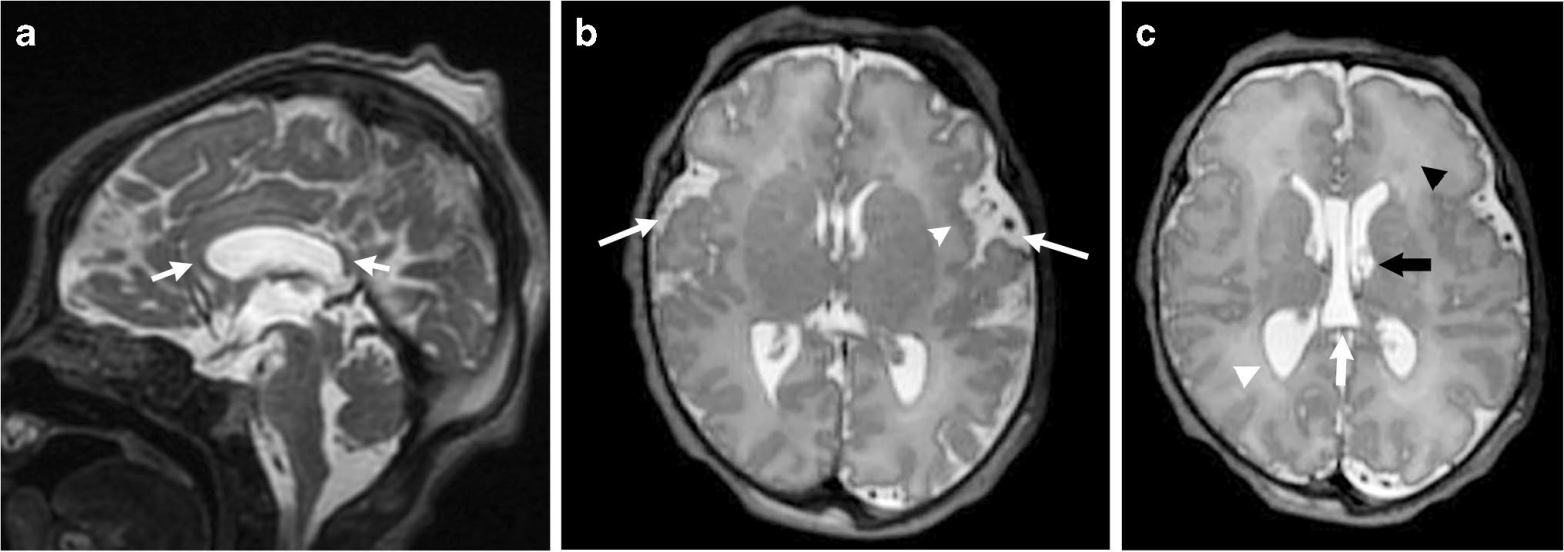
Sotos syndrome in a 4-day-old boy with genetically confirmed *NSD1* gene mutation. a A sagittal T2-weighted image shows a small corpus callosum (*arrows*). Scalp swelling is noted from recent delivery. b An axial T2-weighted image shows widened frontal opercula (*arrows*) and left perisylvian polymicrogyria (*arrowhead*). c An axial T2-weighted image shows ventriculomegaly with large trigones (*white arrowhead*), cavum septum pellucidum and vergae (*white arrow*), and diffusely increased T2 signal within the white matter (*black arrowhead*). Periventricular cysts are also noted (*black arrow*) that could be related to the syndrome or be sequala from previous germinal matrix hemorrhages
